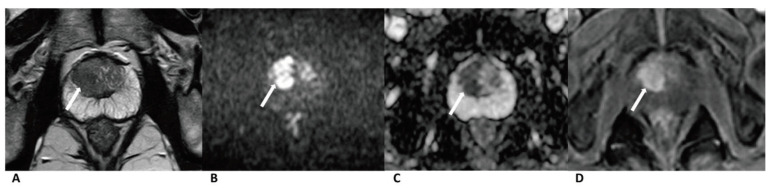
Case of prostate cancer in TZ. Male, 63 years old, prostate cancer with its longest diameter being >1.5cm. T2WI showed a hypointense nodule (arrow) in the right TZ (A). The lesion was hyperintense on DWI (b = 1500 s/mm^2^) (arrow) and hypointense on ADC images (B,C). Positive enhancement was seen on the DCE (arrow) (D). The lesion was finally assessed as a PI-RADS category 5, indicating that it had a high cancer risk according to PI-RADS v2, PA PI-RADS v2, and biparametric MRI protocols.
